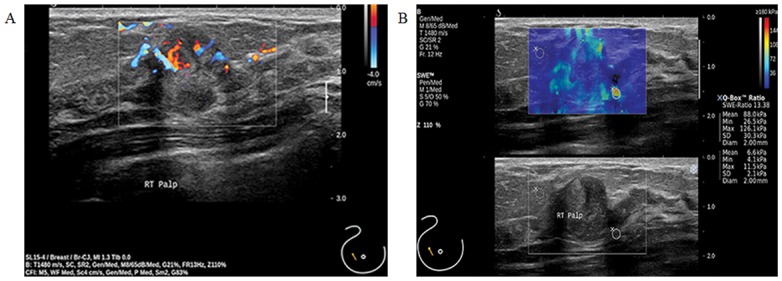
Atypical papilloma of the right breast in a 44-year-old woman. (A, B) This lesion was initially diagnosed a benign papilloma on ultrasound-guided core needle biopsy. This lesion was upgraded after surgical excision. (A) Color Doppler showing increased vascularity in internal portion of mass. (B) The ROI of shear wave elsatography image (top) is set at the margin of the lesion. B-mode ultrasound (bottom) image showing a 1.7-cm sized, irregular shaped and palpable breast lesion considered as category 4B. Adjacent ductal dilatation is also seen.
